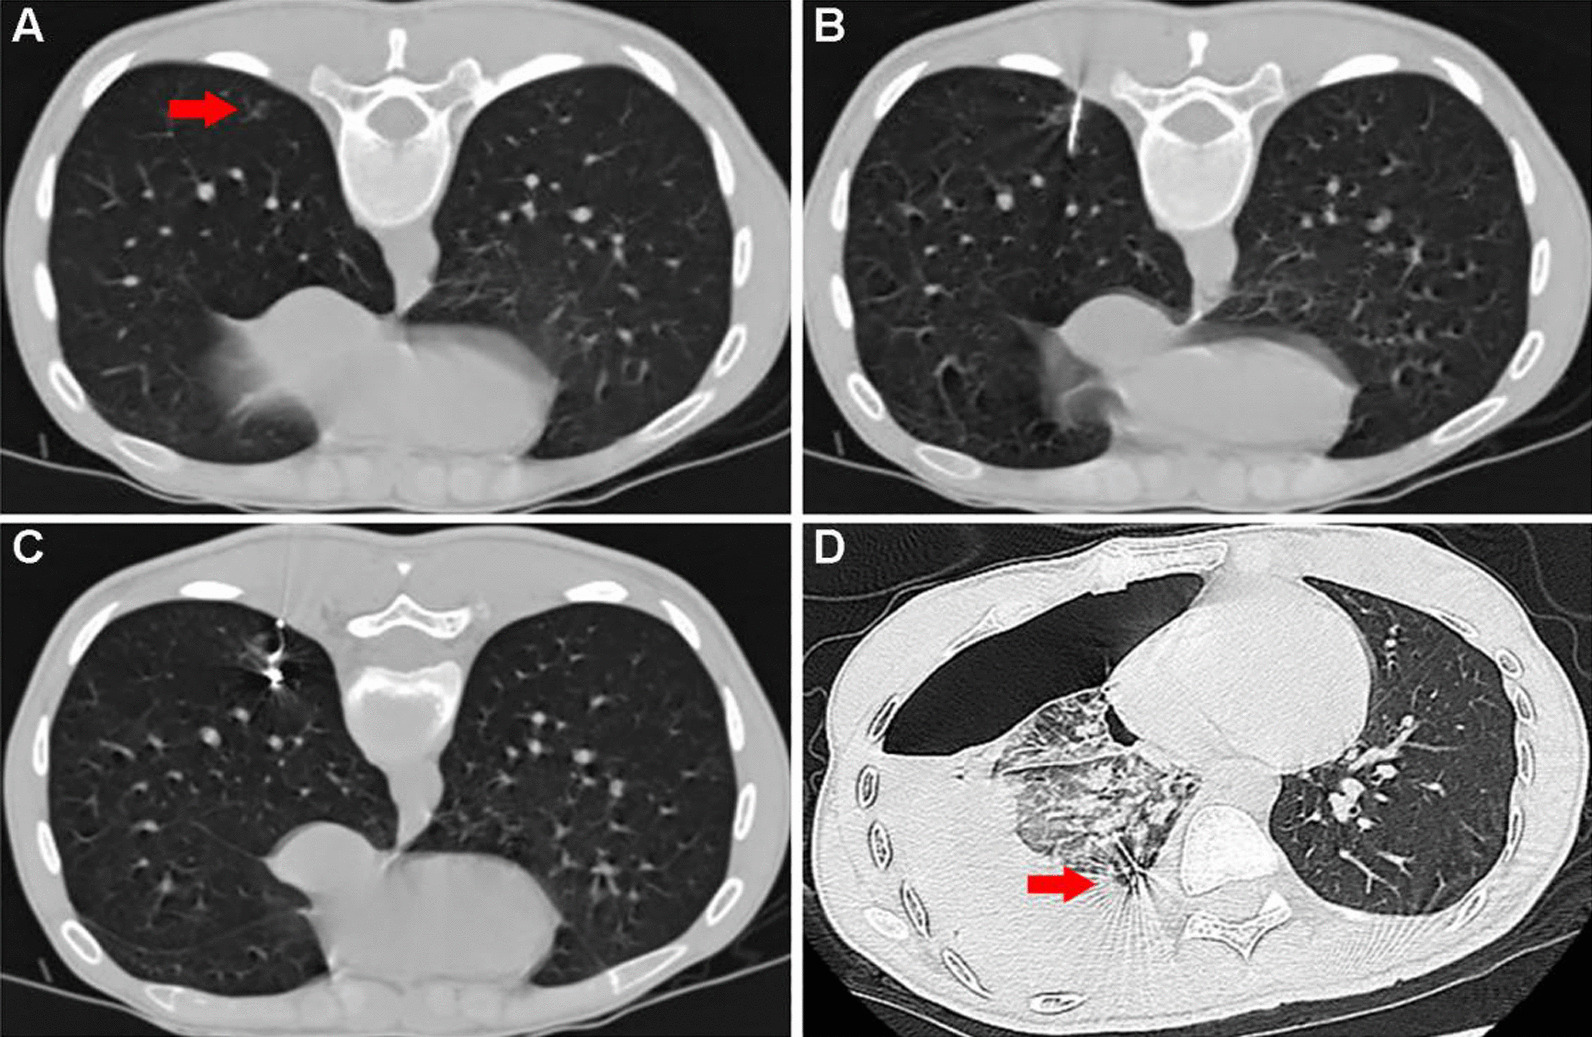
A The location of the ground glass nodule (red arrow) in prone position. B The tip of the introducer needle was placed adjacent to the edge of the nodule. C The distal end of microcoil was placed next to the nodule and the proximal end was left on the parietal pleura. No obvious pneumothorax was seen. D Emergency chest CT reveals massive hydropneumothorax and right lung collapse at 10 h after the localization. The microcoil can still be found in the lower right lung (red arrow)
